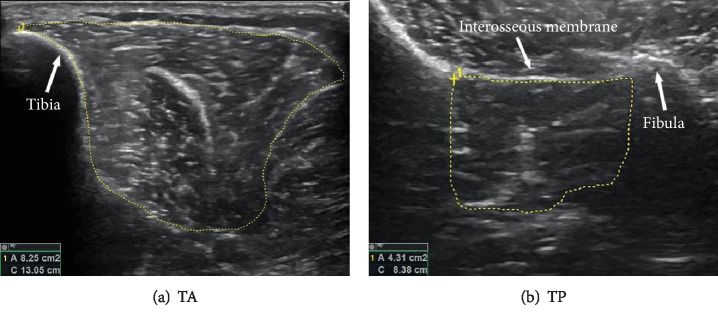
Ultrasound images showing TA (a) and TP (b) measurement borders. The tibia and fibula were used as landmarks with the interosseous membrane in full view to capture the entire circumference of the muscles (yellow outlines).
